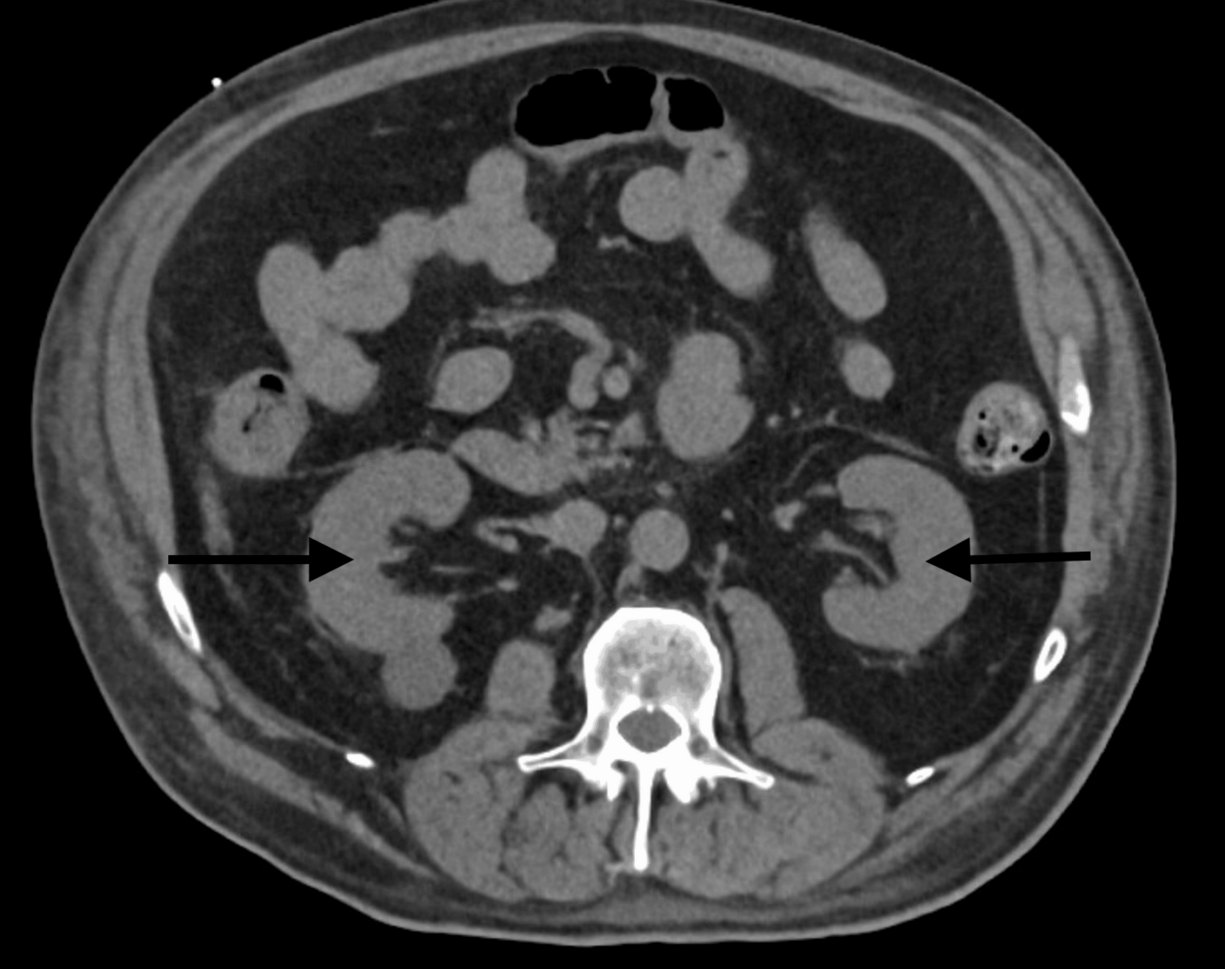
Axial Non-contrast CT of the Abdomen - Kidneys The CT image demonstrates both kidneys with preserved size and contour, without hydronephrosis, obstructing stones, or masses (black arrows).
